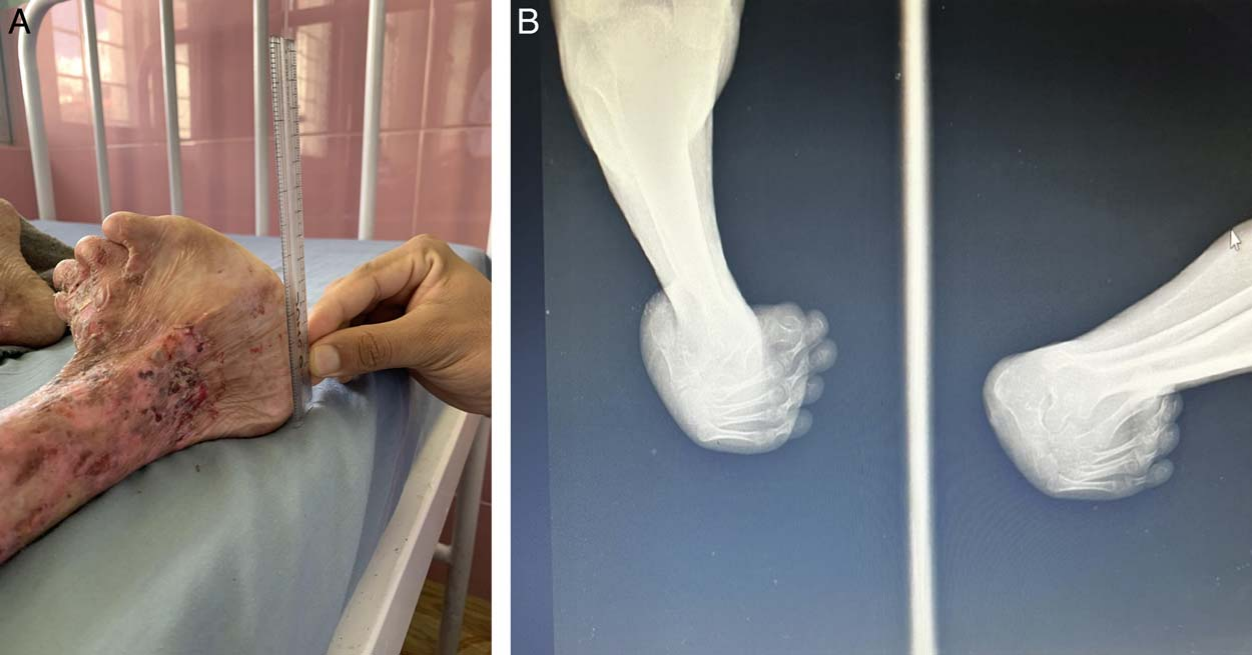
(A) Absence of nails with right limb shortening, adductus, and varus deformity; (B) X-ray showing right limb adductus and varus deformity.
